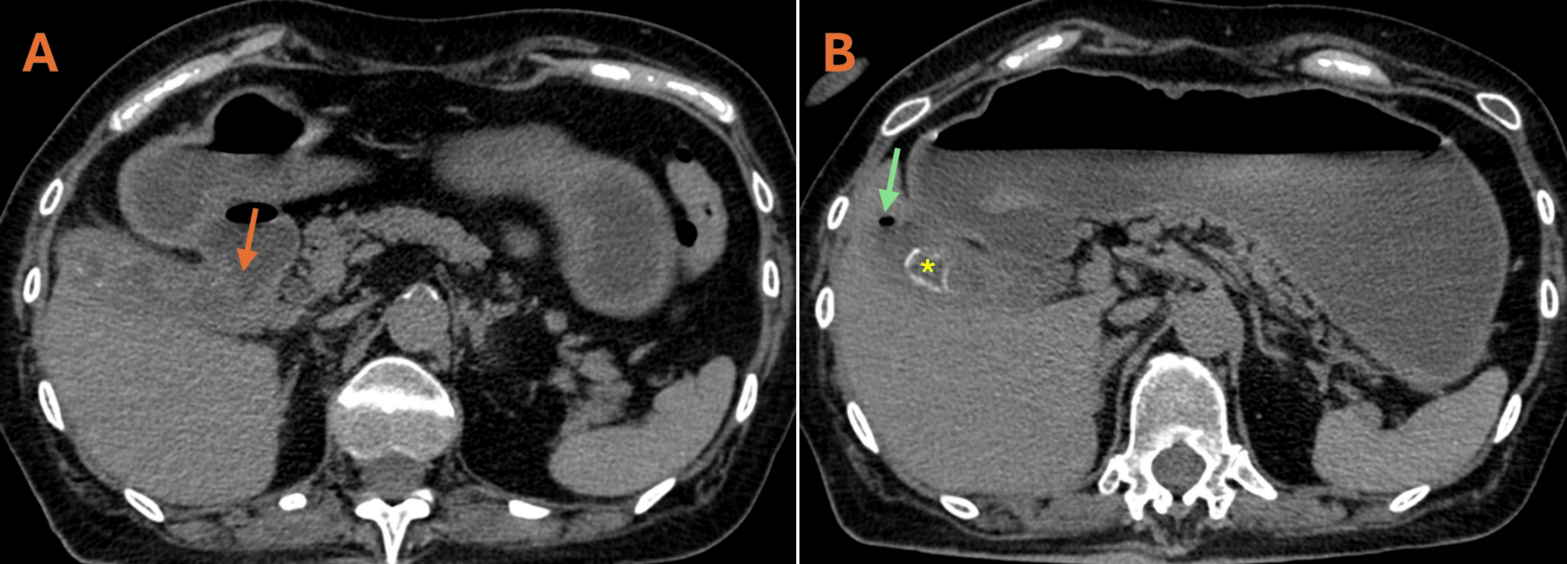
Cholecystoduodenal fistula. Axial CT slices without contrast. In image A, there is a lack of definition of the anterior wall of the gallbladder body and the first portion of the duodenum, suggesting a cholecystoduodenal fistula (orange arrow). In image B, the presence of mineralized calculus (green arrow) and gas (asterisk) in the gallbladder lumen can be identified. CT: computed tomography
